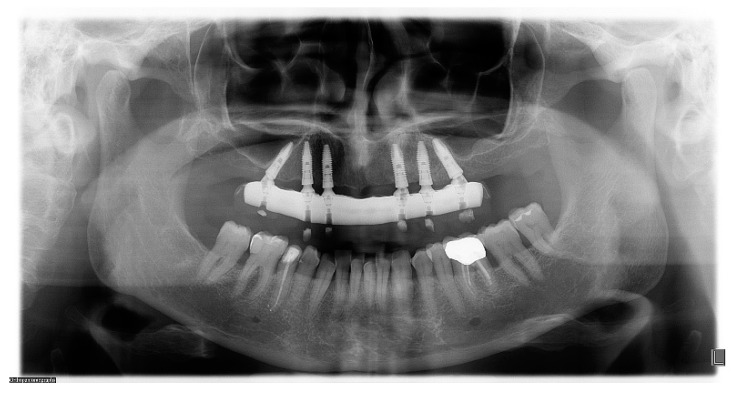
Panoramic radiograph at follow-up.
